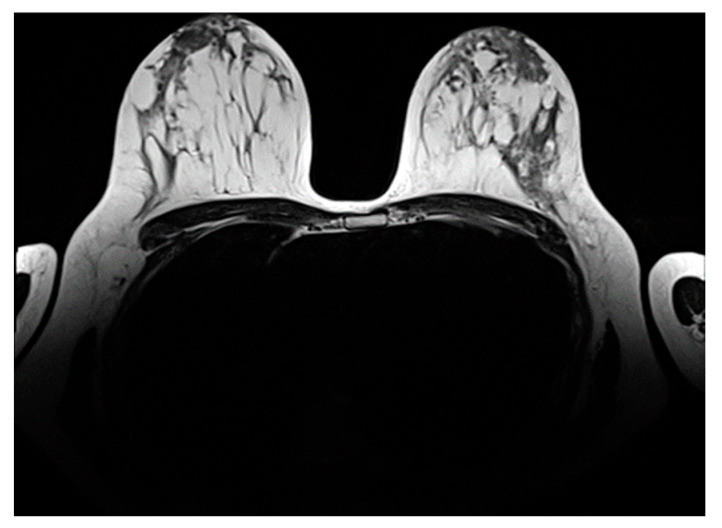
Post treatment control MRI T2 (institutional patient data).
